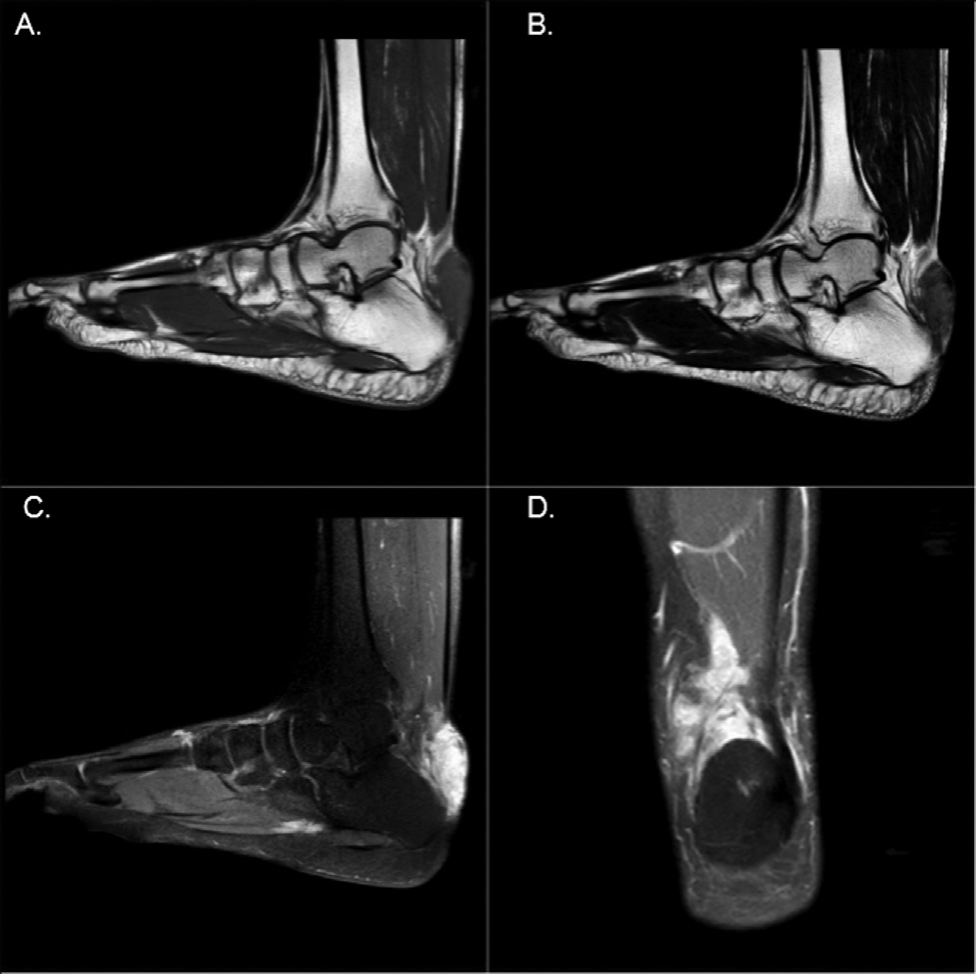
MR image of the right foot and ankle showing (
